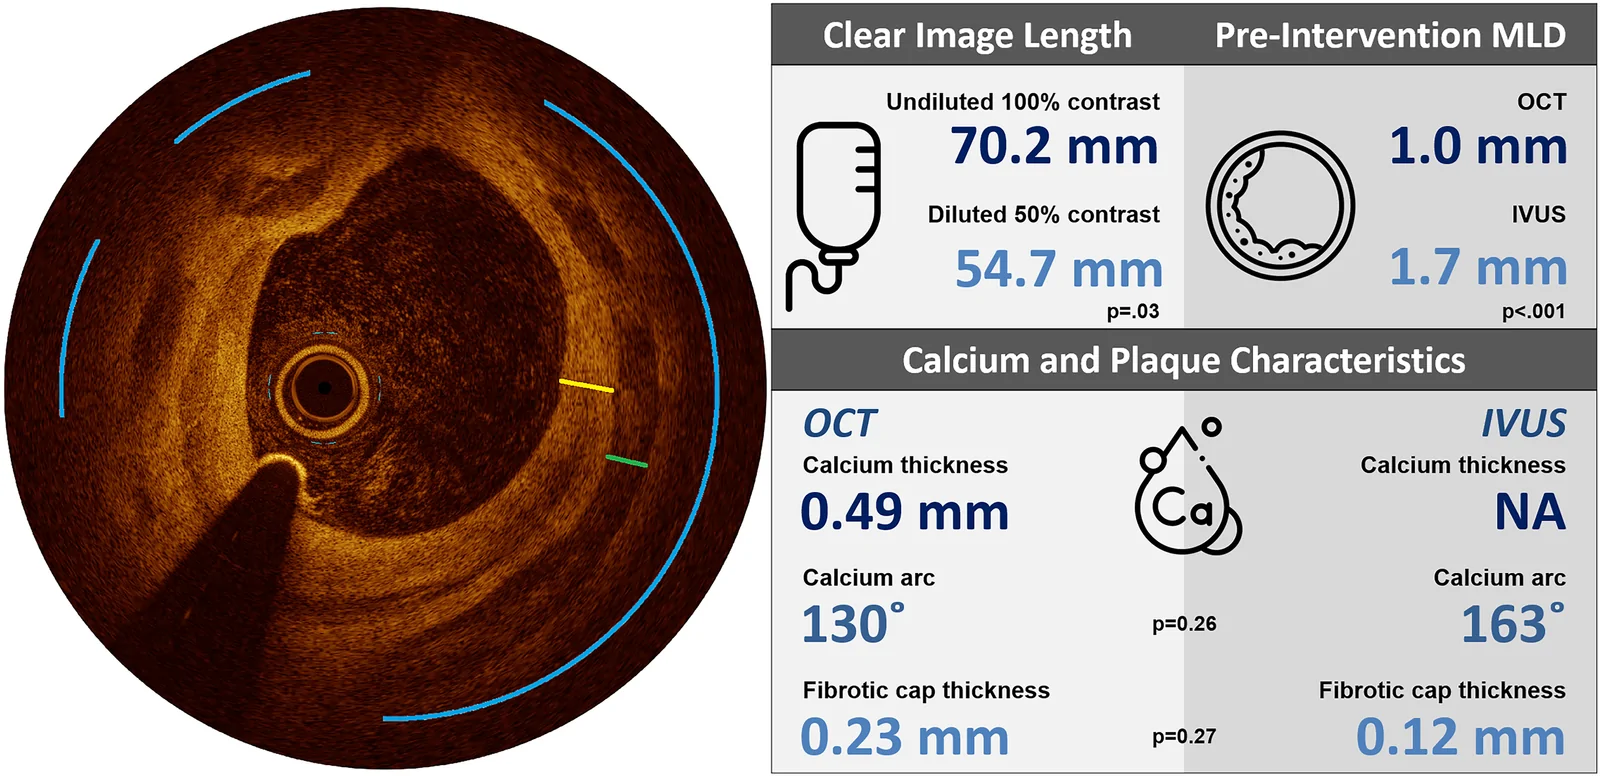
Calcium analysis by optical coherence tomography (OCT) is shown in the left panel, with blue denoting the arc of calcium, which is incontiguous in this example. The fibrotic cap thickness and calcium thickness are designated by yellow and green lines, respectively. Clear image length analysis is congruent with undiluted contrast, providing better imaging quality than diluted, 50% contrast on OCT imaging (*P* = .028). Preintervention minimum lumen diameter (MLD) was lower by OCT compared to intravascular ultrasound (IVUS) (*P* < .001), likely owing to mechanistic differences in image acquisition in the 2 modalities, whereby the former measures intraluminal diameter and the latter measures external elastic lamina. Calcium and fibrotic plaque characteristics were not different between the 2 intravascular imaging modalities, though OCT is capable of capturing calcium thickness, whereas IVUS is unable to penetrate through calcium to measure the depth of involvement. NA, not applicable.
